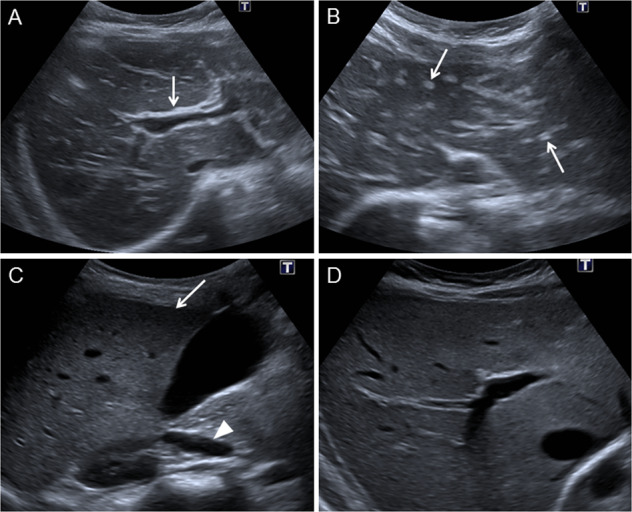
Liver ultrasound (US) images showing hepatic findings of a patient with the POLG1 p.G517V variant. Liver US performed at week 86 during maintenance therapy showed increased periportal echogenicity in the main portal vein (A, arrow) and segmental portal branches (B, arrows). Liver US performed at week 90 demonstrated regression of periportal echogenicity (C), but it showed mild hyperechogenicity of liver parenchyma (arrow) compared with that of the kidney (arrowhead). Follow-up US one year after the first US still showed slight hyperechogenicity of liver parenchyma (D).
